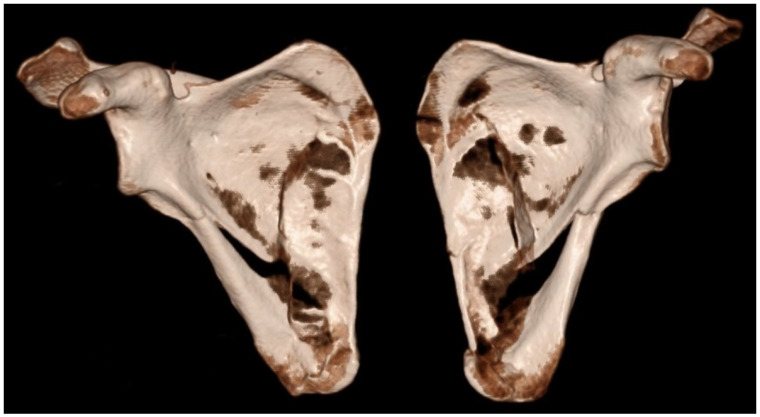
3D CT reconstruction showing bilateral fractures of the scapular body and spine (anteroposterior view).
